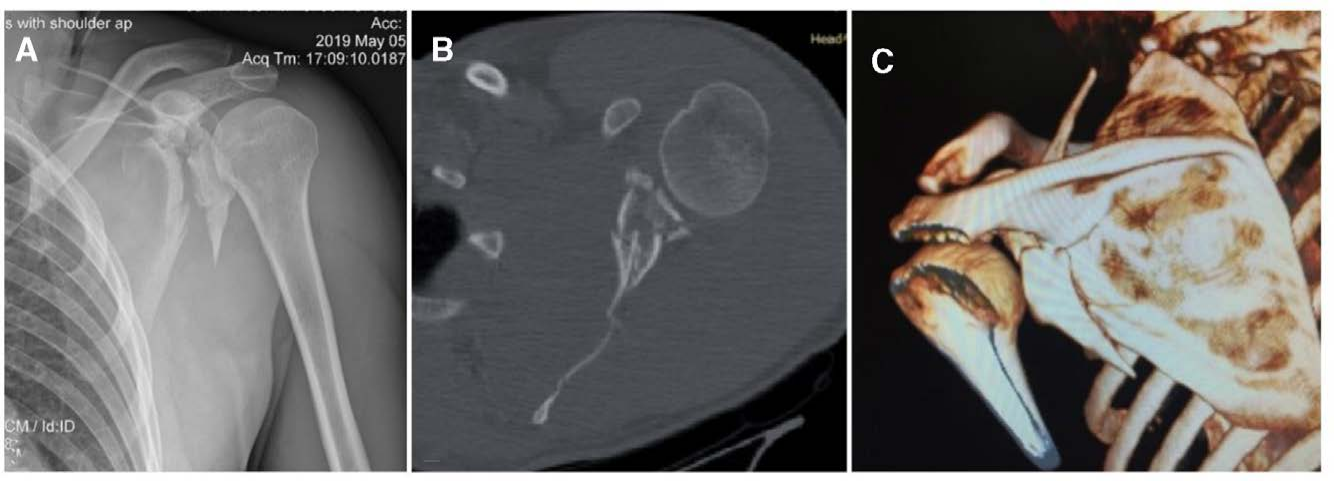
(A) Anteroposterior X-ray of the left shoulder, (B) CT axial view, (C) 3D CT reconstruction.
